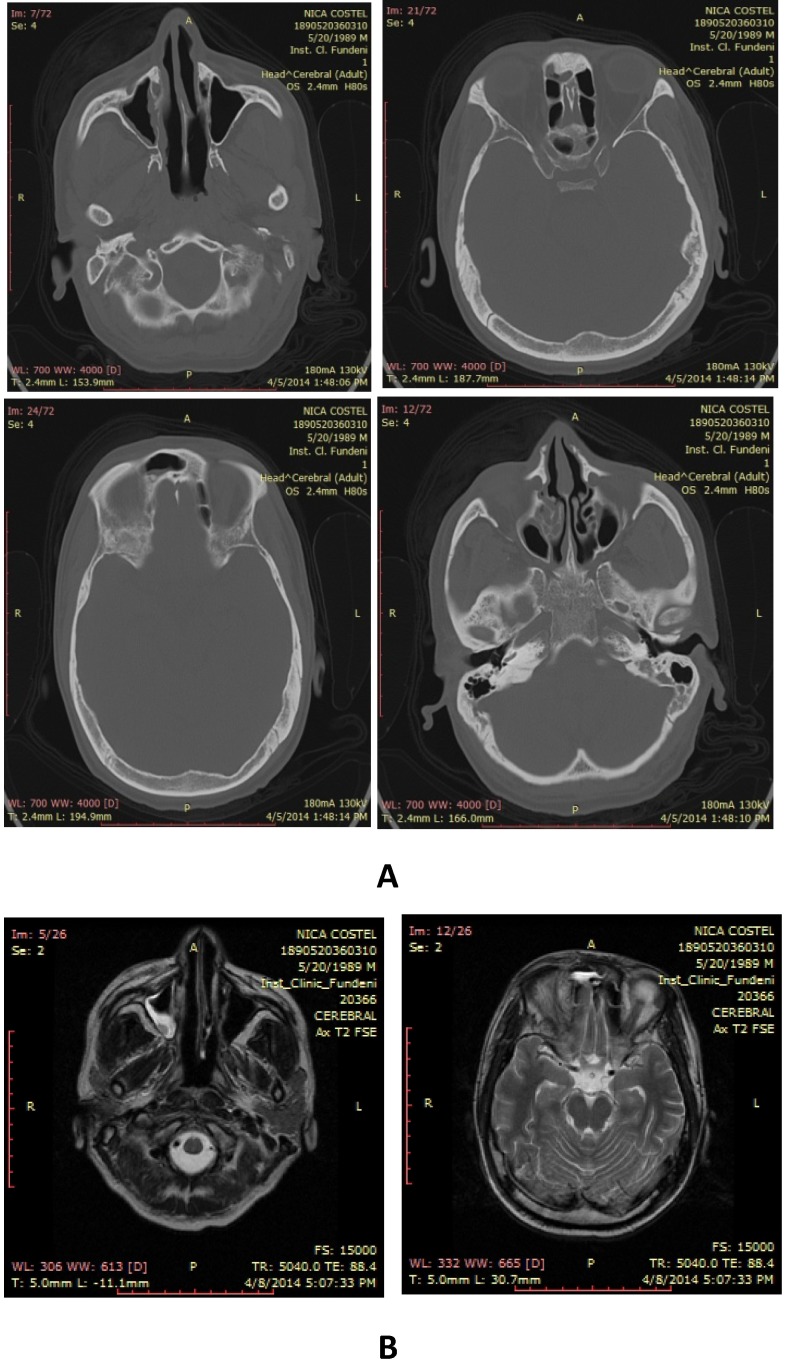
Cerebral MRI A) Mucosal thickening of the posterior wall of the right frontal sinus, ethmoidal cells of right maxillary and bilateral sphenoid sinuses; B) Symmetrical mucosal thickening of paranasal sinuses, moderate right periorbital edema, minor right intraorbital adipose tissue edema, secondary exophthalmos
